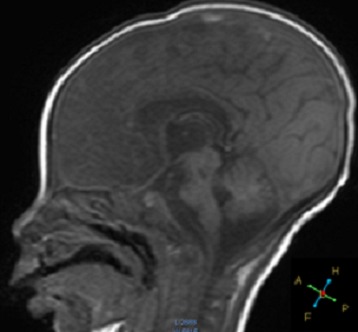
MRI of the patient, sagittal image: it was not possible to identify the Pituitary
